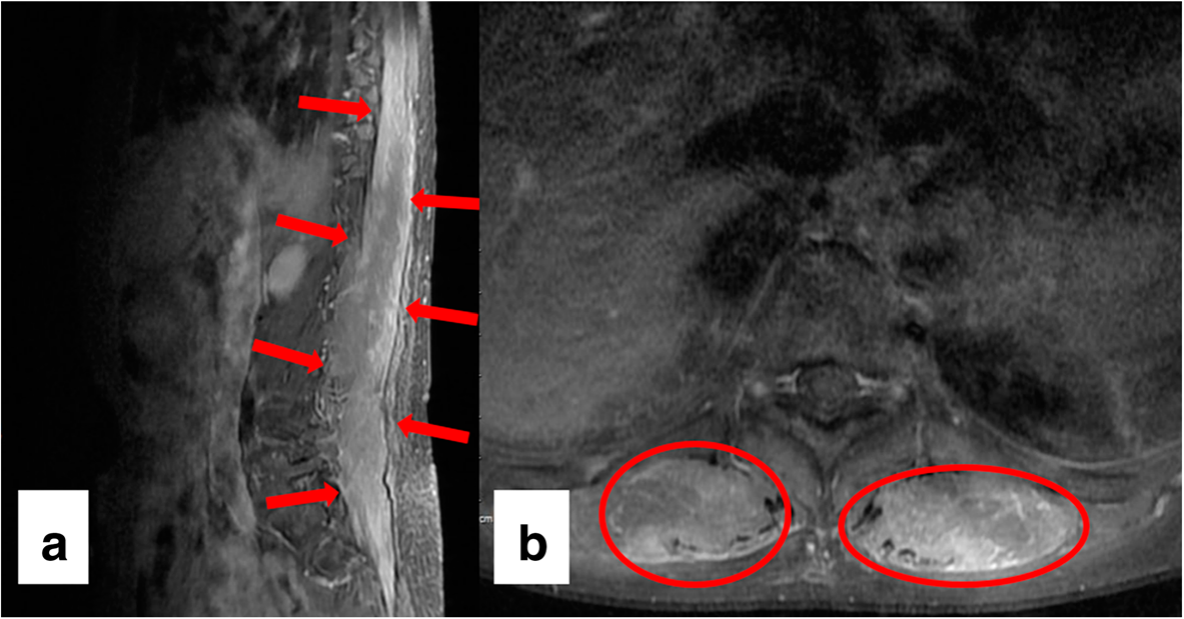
Spine MRI with gadolinium contrast taken three months after the onset of camptocormia. a Sagittal T1-weighted imaging reveals diffuse enlargement and patchy enhancement of the paraspinal muscles (*red arrows*) through the thoracic, lumbar, and sacral spine. b Axial T1-weighted imaging shows bilaterally increased signal intensity of paraspinal muscles (*red circles*) at the level of T10
